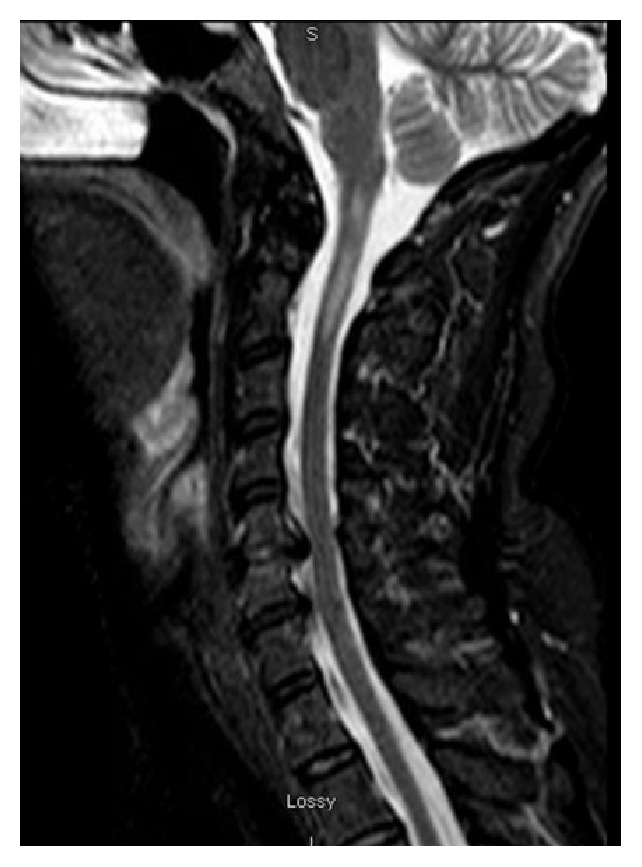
MRI cervical spine T2 sagittal sequence showed a hyperintensity extending from C1 to C2.
